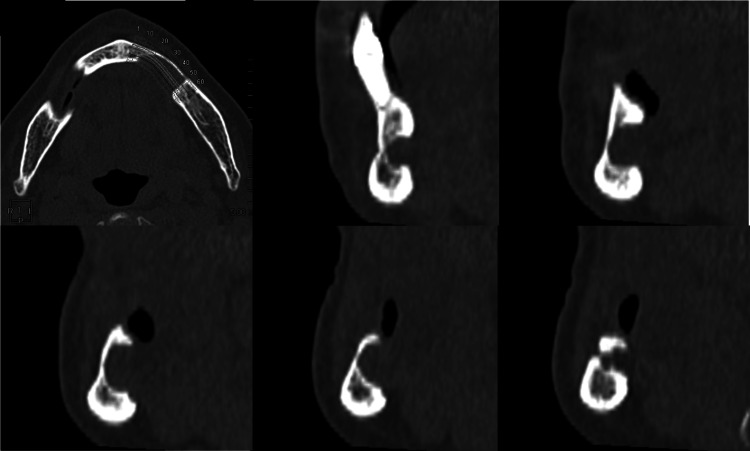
Multidetector CT image (hard tissue mode). A single-vesicular cavity is observed, possessing a cortical margin and opening toward the lingual side. The margin is relatively distinct, sharp, and regular.
